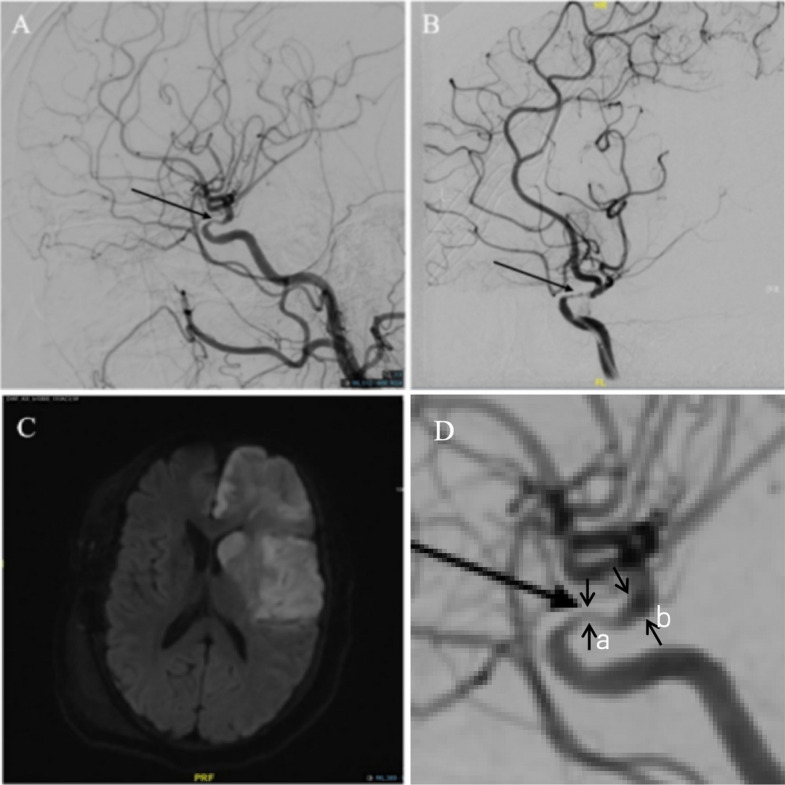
Infarction of a major artery with an aggravation of severe stenosis. DSA and MR images of a 40-year-old female patient with MMD show aggravated stenosis of the terminus of the left ICA (arrow in (A), before the operation; arrow in (B) after the operation) and new infarction of the ICA area (C, diffusion-weighted imaging after recanalization). D Magnified view of a part of the image in (A), showing the method used to assess the severity of stenosis in major arteries: comparison between the minimum diameter (a) at the narrowest segment and the maximum diameter (b) of the distal segment
